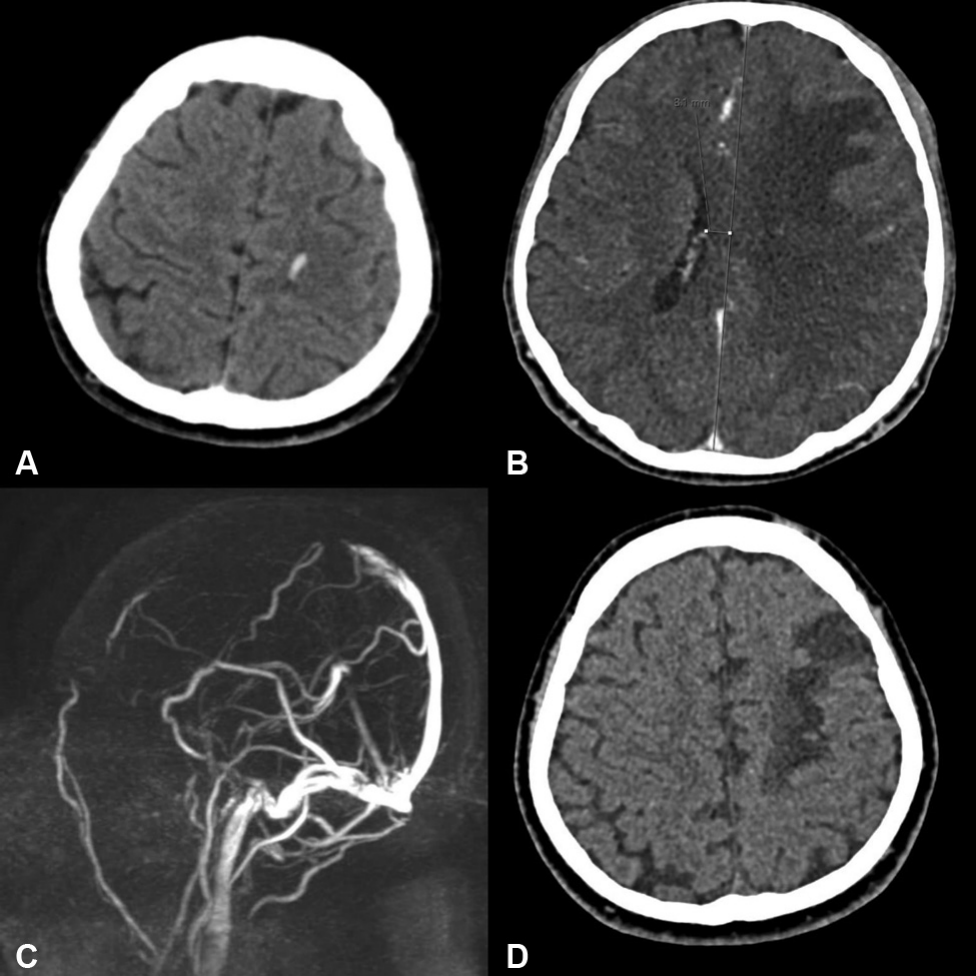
Example of herniated case with juxtacortical hemorrhage. On uncontrasted cranial CT, (
